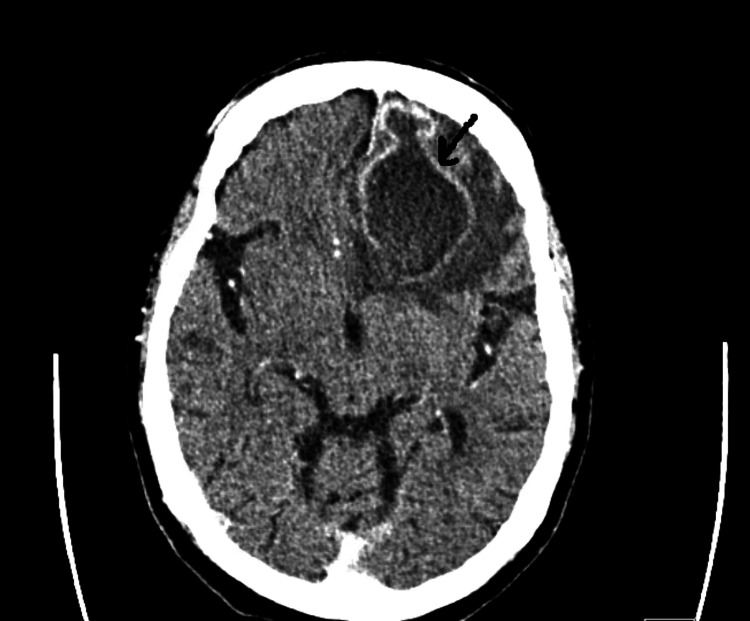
CT of the head with contrast CT of the head with contrast revealed a peripherally enhancing mass in the left frontal lobe.
